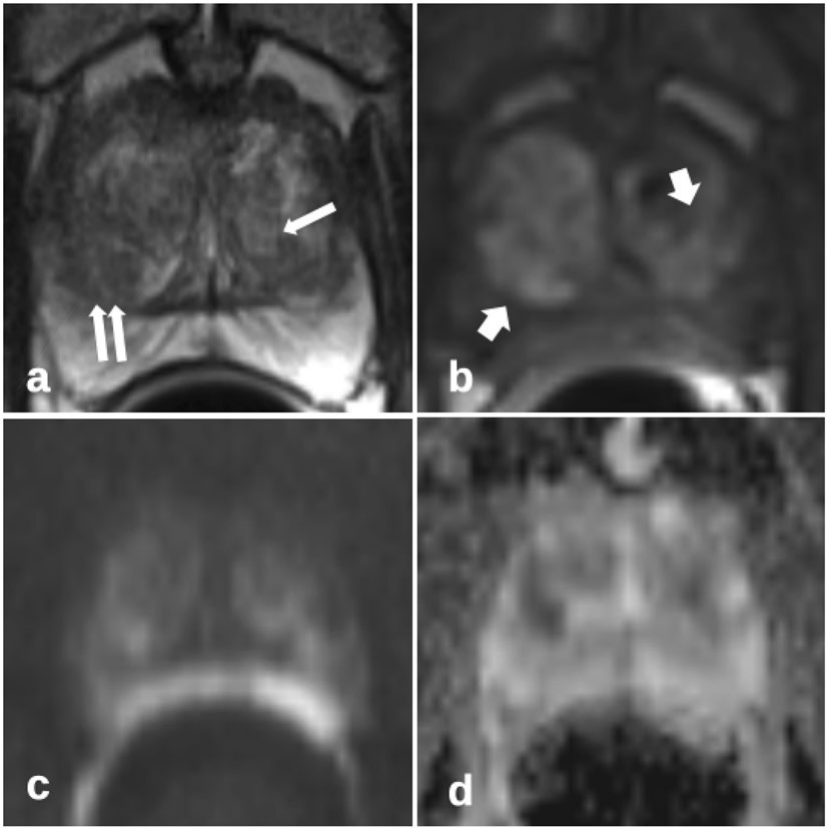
Unspecific heterogeneous perfusion pattern (short arrows in b) of the transition zone in benign prostate hyperplasia, without marked asymmetry and marked high signal intensity; patient of 57 years, PSA 5.1 ng/ml, prostate volume 57 ml, no carcinoma; a T2-weighted imaging axial with marked biopsy traces (long arrows); b T1-weighted early-phase dynamic perfusion; c diffusion-weighted imaging with b = 1400; d apparent diffusion coefficient map
